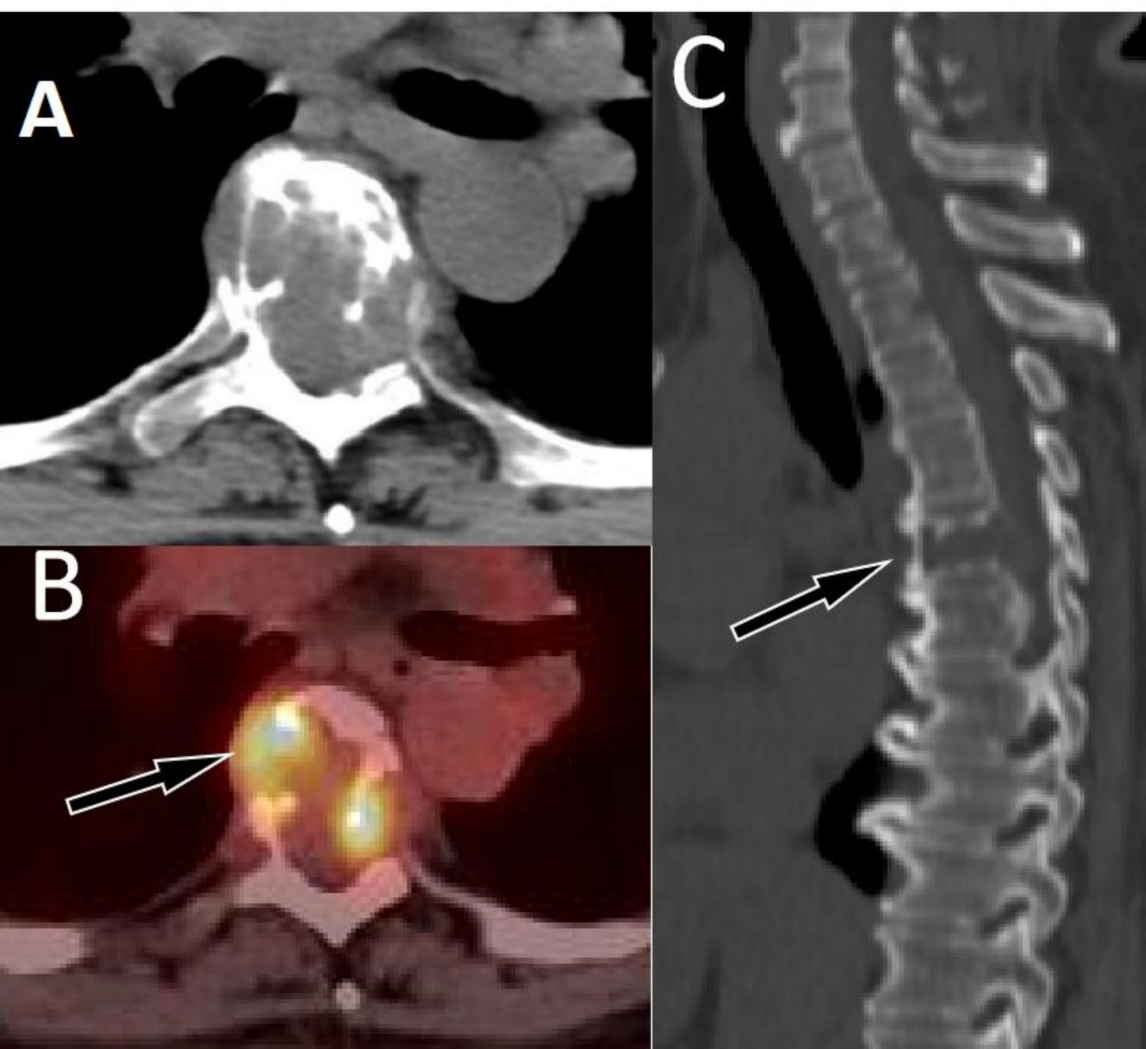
A 51-year-old woman with EHE; (A) Axial CT revealed low-density osteolytic bone destruction shadow in the 6th thoracic vertebral body (arrow), which broke through the bone cortex and formed a soft tissue mass in the surrounding area; (B) The PET/CT fusion image of the corresponding area shows mildly increased ^18^F-FDG uptake (arrow), with a SUVmax of 6.0; (C) Sagittal CT reveals compressive changes in the vertebral body, with low-density cavity changes in the neutral state of the vertebral body (arrow), without sclerotic rims.
